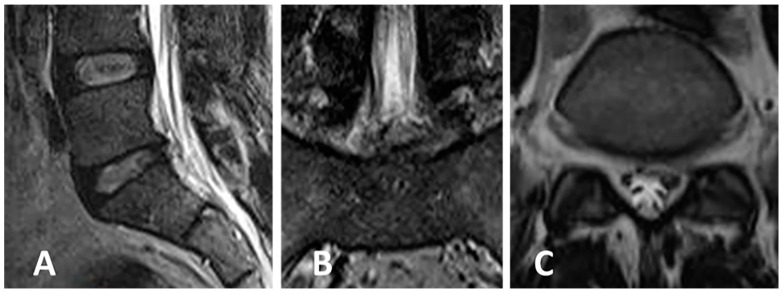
(A–C) Postoperative MRI (of suboptimal quality since the patient was restless during examination) shows adequate epidural decompression after removal of the herniated disc with the TESSYS technique. VAS leg 0, ODI 4.
